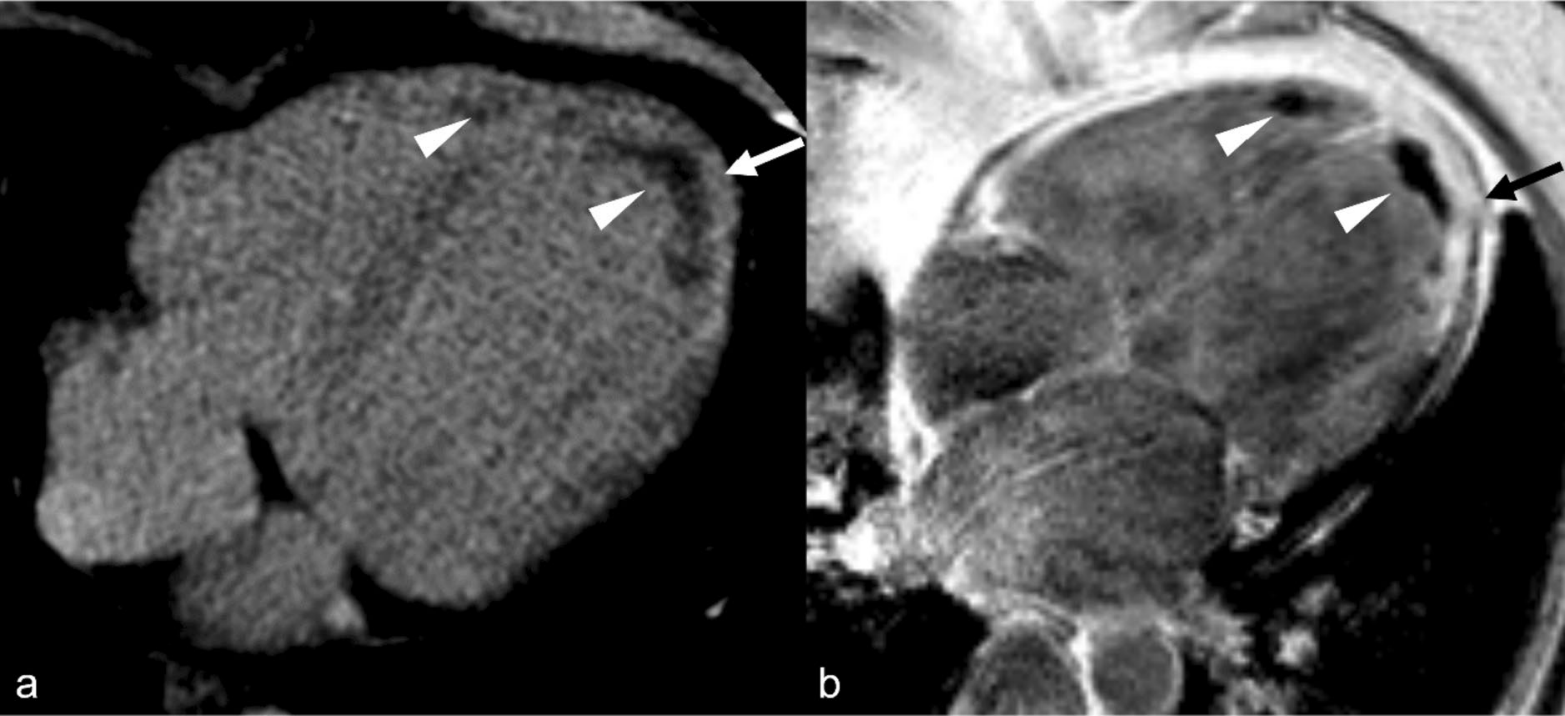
Late iodine enhanced (LIE) CT image reconstructed in four chamber view (a) and late gadolinium enhanced (LGE) image acquired on the corresponding plane during a cardiac-MR (b) in a patient with an acute myocardial infarction of the left circumflex artery territory. In both images is evident the contrast enhancement of the infarcted myocardium (white and black arrows) and the thrombi adherent to the apex in both ventricles (white arrowheads)
